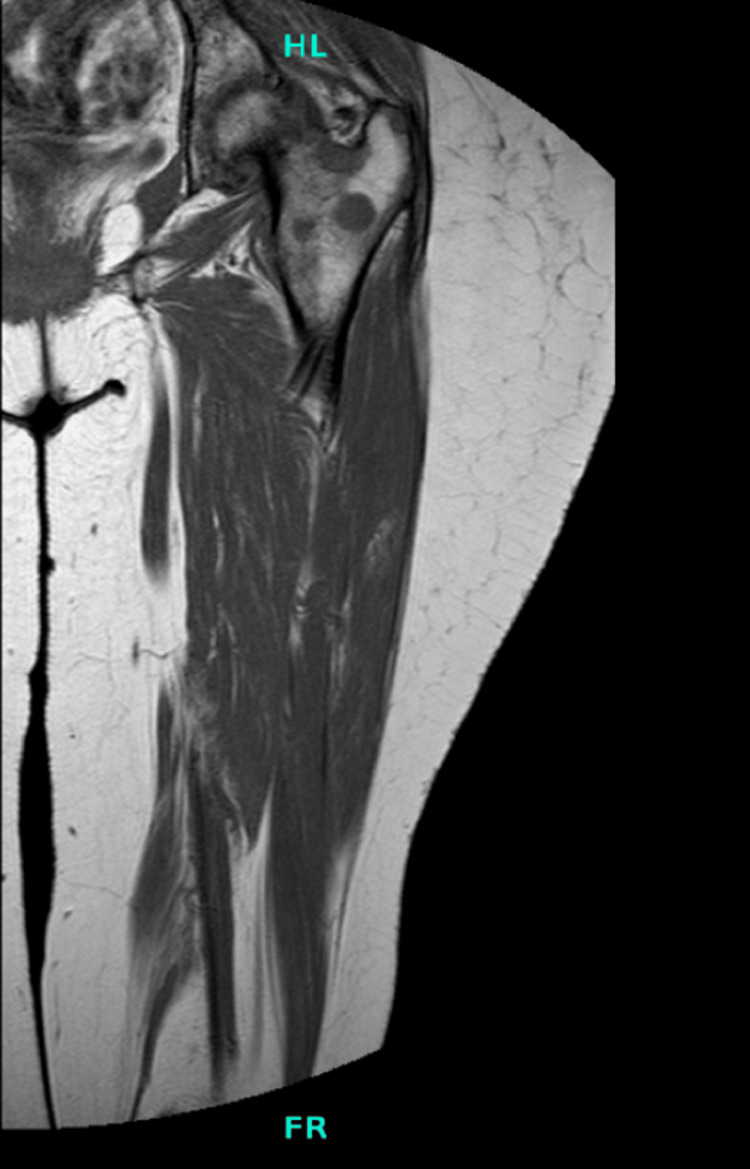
MRI of left hip demonstrating peritrochanteric lesions MRI: magnetic resonance imaging
